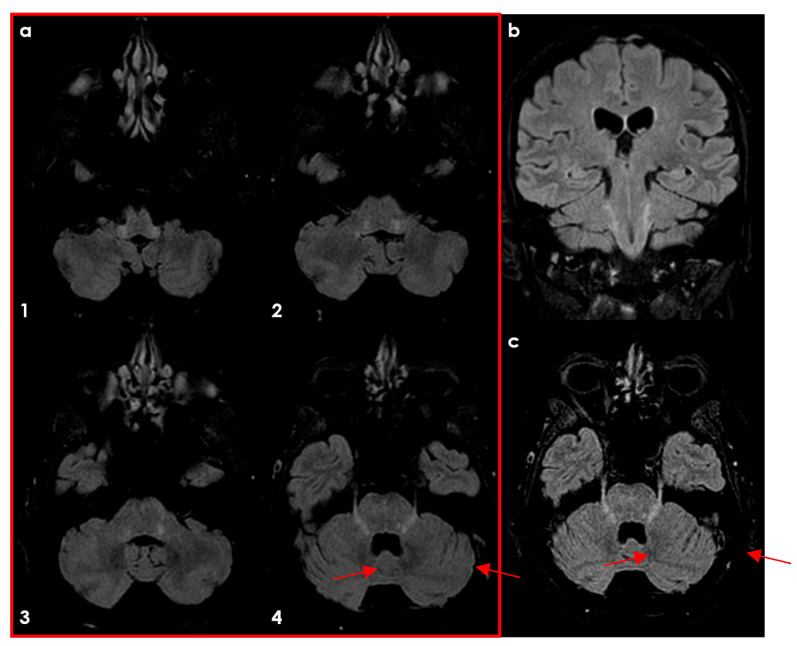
Axial FLAIR MRI in a patient with multiple sclerosis and trigeminal symptoms on the right side, showing, in ascending slices, panel (a1–a4), hyperintense signal in both trigeminal tract and a bilateral trigeminal pontine sign (red arrows). In panel (b), the coronal FLAIR is proposed, showing the longitudinal extension of pontine hyperintensities. In the panel (c), the axial oblique FLAIR slice, focused on the plane of cisternal TN, is reconstructed, showing a bilateral hyperintensity of both TN and intrapontine segment (red arrows).
